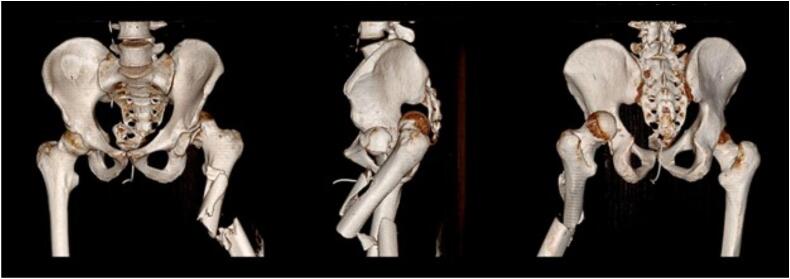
Pre-operative assessment of injuries by computed tomography imaging using 3D reconstruction.
